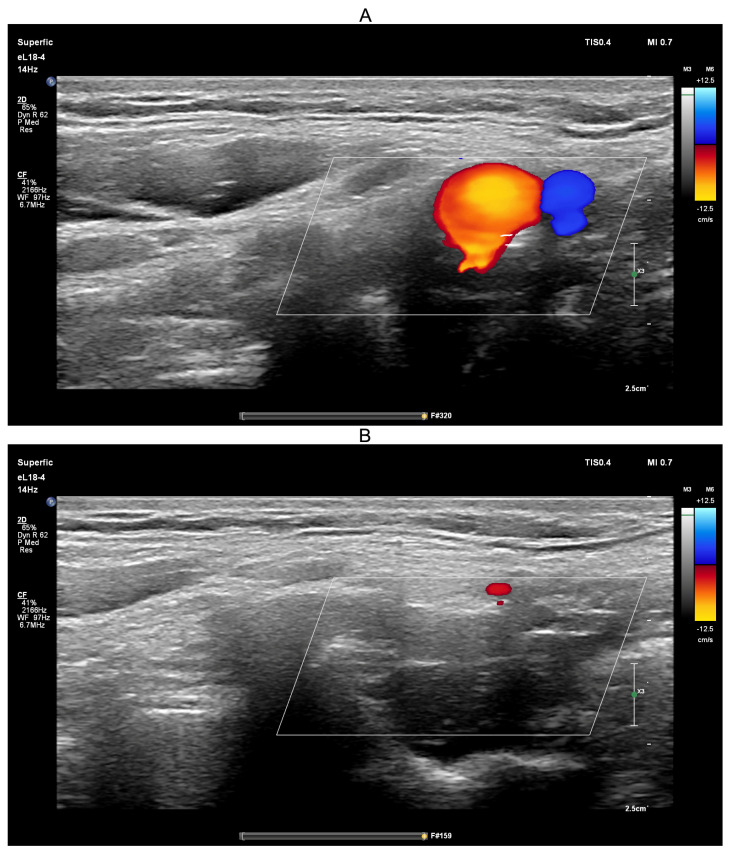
Screenshots from the PHILIPS EPIQ Elite ultrasound triplex scanner during the ultrasound-guided common carotid artery compression test (transient hypoemic/hyperemic response test). (A)—Before compression of the common carotid artery; (B)—During compression of the common carotid artery.
